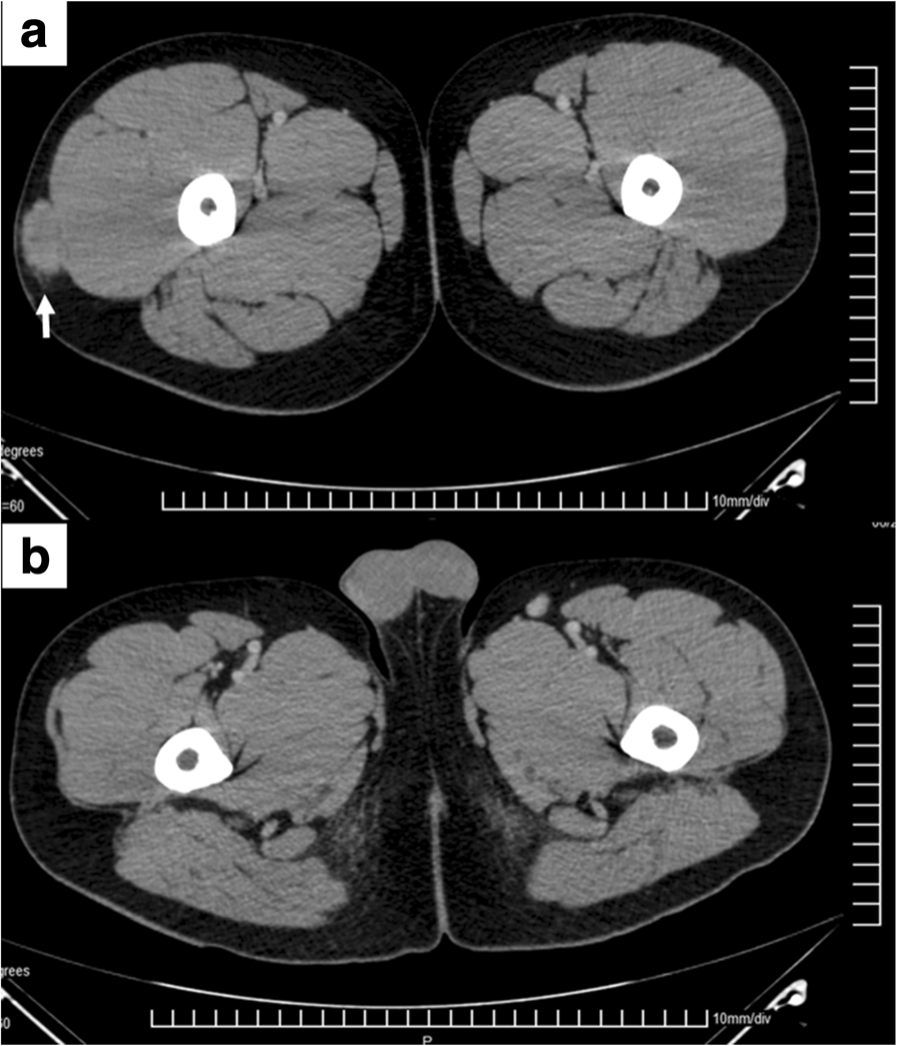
a Axial CT image of the inferior extremities shows a muscle-density mass in the lateral aspect of the right thigh, measuring 38 × 20 mm (arrow). b Axial CT image of the perineum at the testes level shows the lack of disease in these structures
